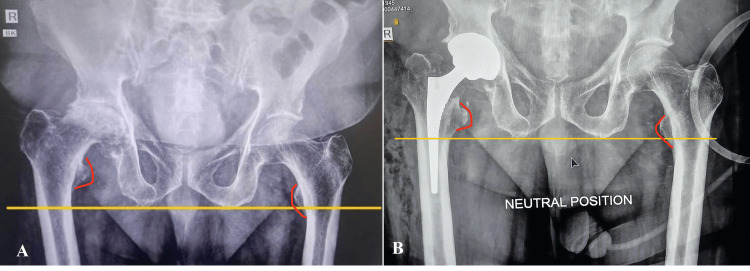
Radiographic evaluation of limb length discrepancy (LLD) following direct anterior approach total hip arthroplasty Panel A: Preoperative anteroposterior pelvic radiograph of a patient with significant limb length discrepancy (LLD, 2.6 cm) due to advanced hip pathology. The yellow reference line indicates the baseline limb length difference, and red curved lines show the difference in lesser trochanter position. Panel B: Immediate postoperative radiograph after total hip arthroplasty via the direct anterior approach showing partially corrected LLD to 1.5 cm postoperatively (yellow line) with the hip in a neutral position, with the lesser trochanter (red curved lines) as a point of reference.
